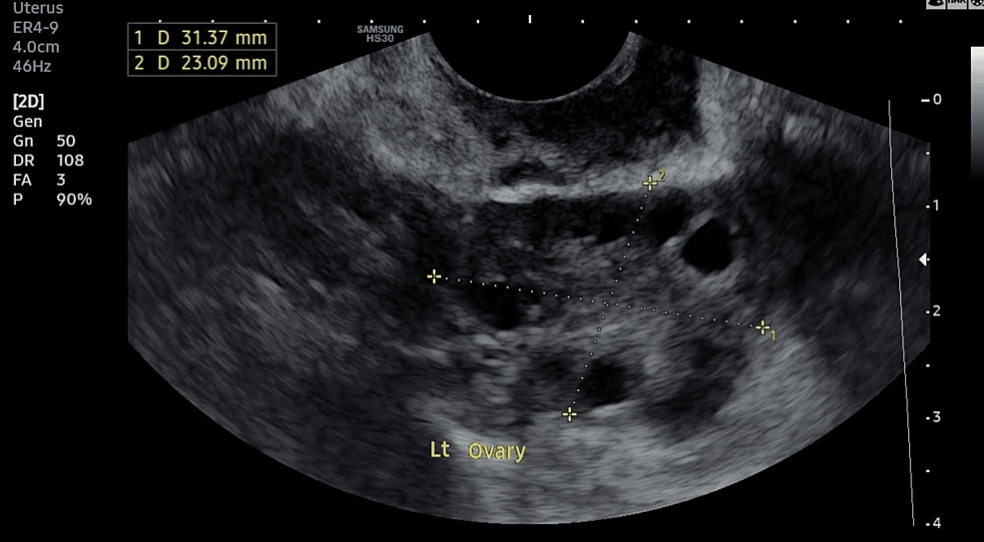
Left (Lt) ovary showing eight follicles on day 3 via transvaginal sonography
